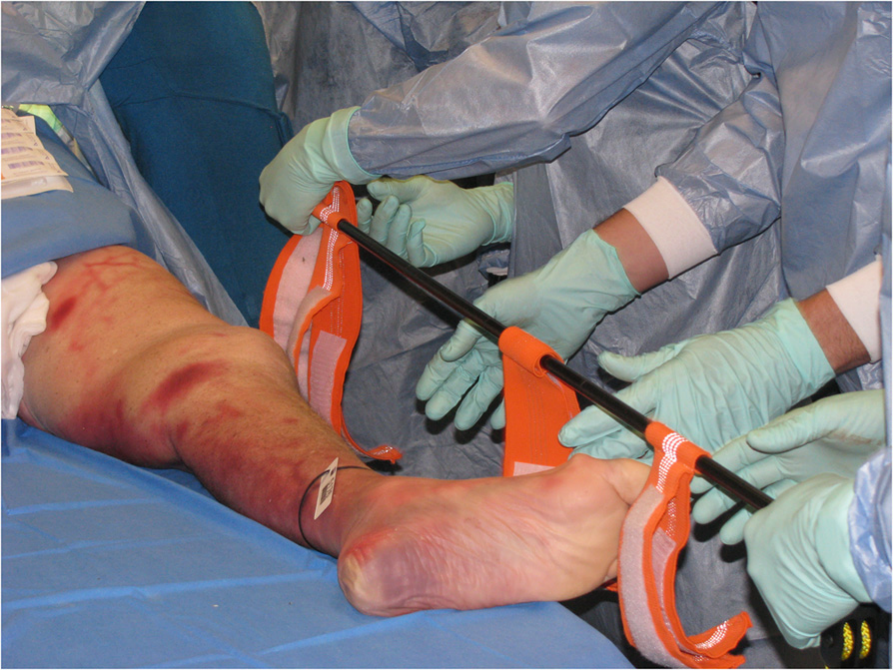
Students performing CT-6 traction splint. Consent for the photographs was obtained from students and representative of the donor.
